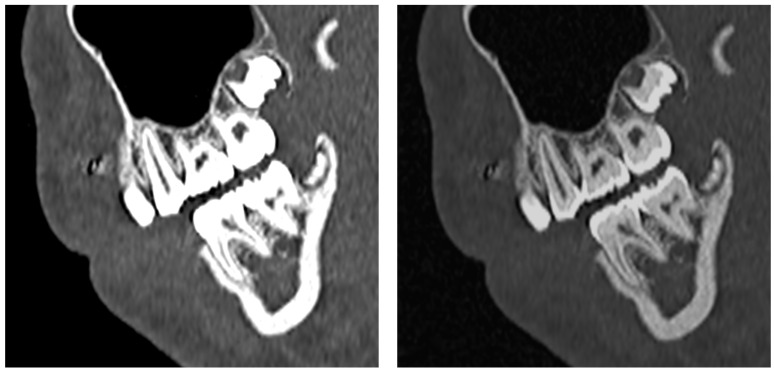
Sagittal section CT shown in bone window and level (left). On the right, the window has been widened and the level has been increased to allow demonstration of the enamel and dentin components of the dentition.
